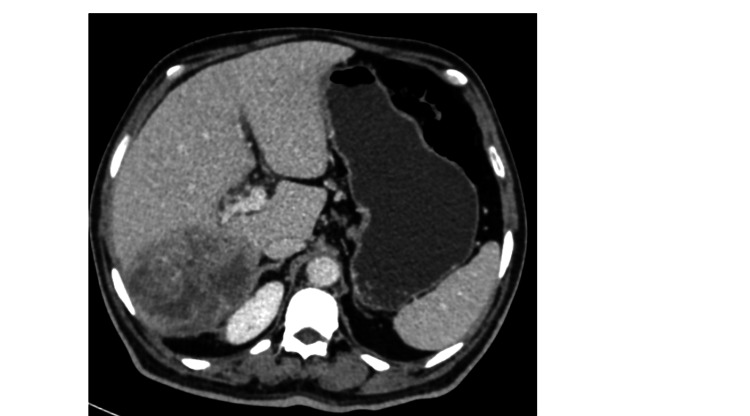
CECT images showing interval regression of the tumor CECT: contrast-enhanced computed tomography
